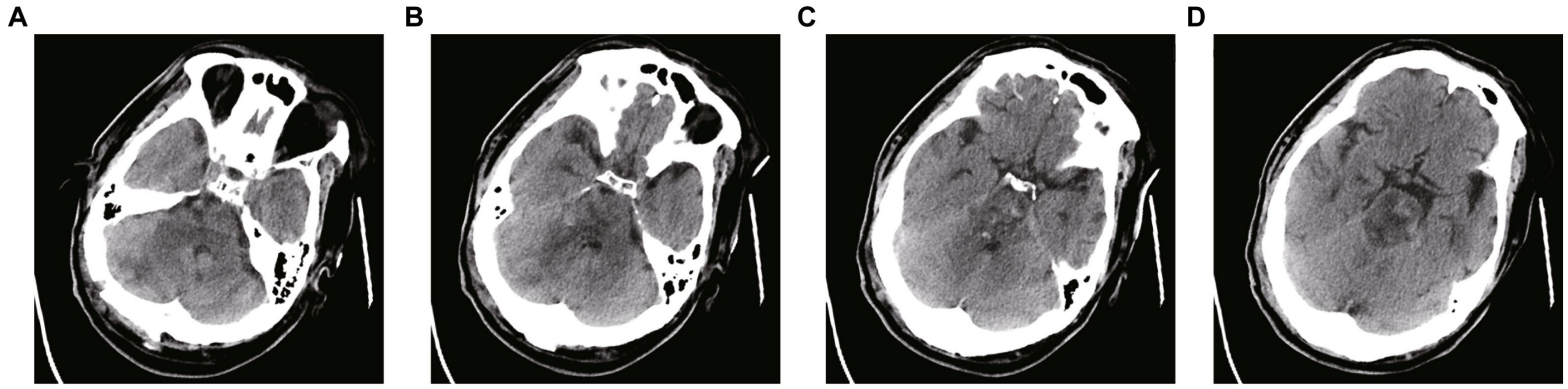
(A–D) The follow-up CT scans 70 h after surgery showed the hematoma was nearly completely removed and the drainage tube had been extracted.
